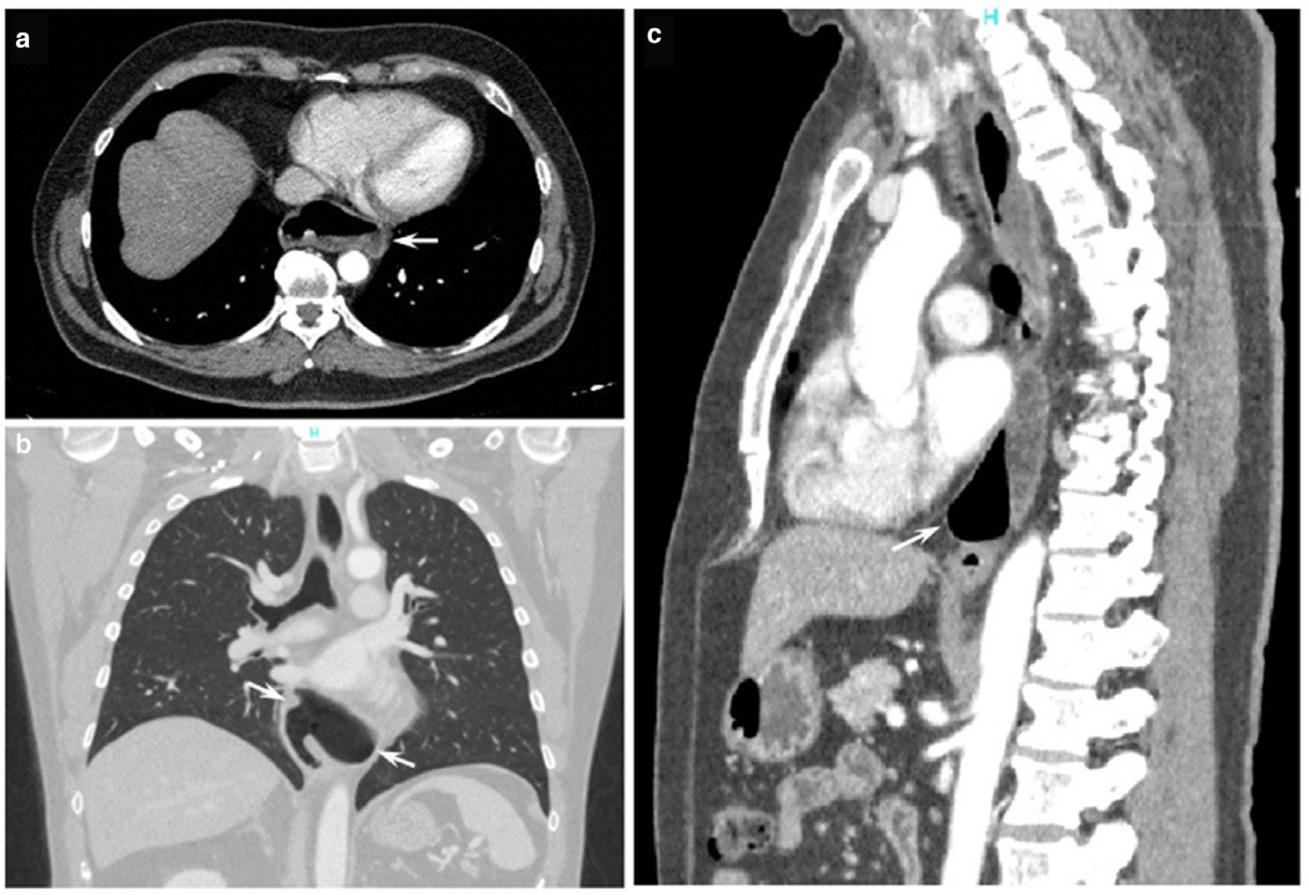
Pre-operative CT scan: June 2021. (A) Axial CE CT chest image in soft tissue window demonstrated a large outpouching on the left wall in the lower esophagus (arrow), with air-fluid level, due to the large esophageal diverticulum; (B) coronal CE CT chest image in lung window clearly showed the large left-sided esophageal diverticulum communicating with the esophagus (arrow); (C) sagittal CE CT chest in soft tissue window image showed the retrocardiac extension of the diverticulum (arrow).
